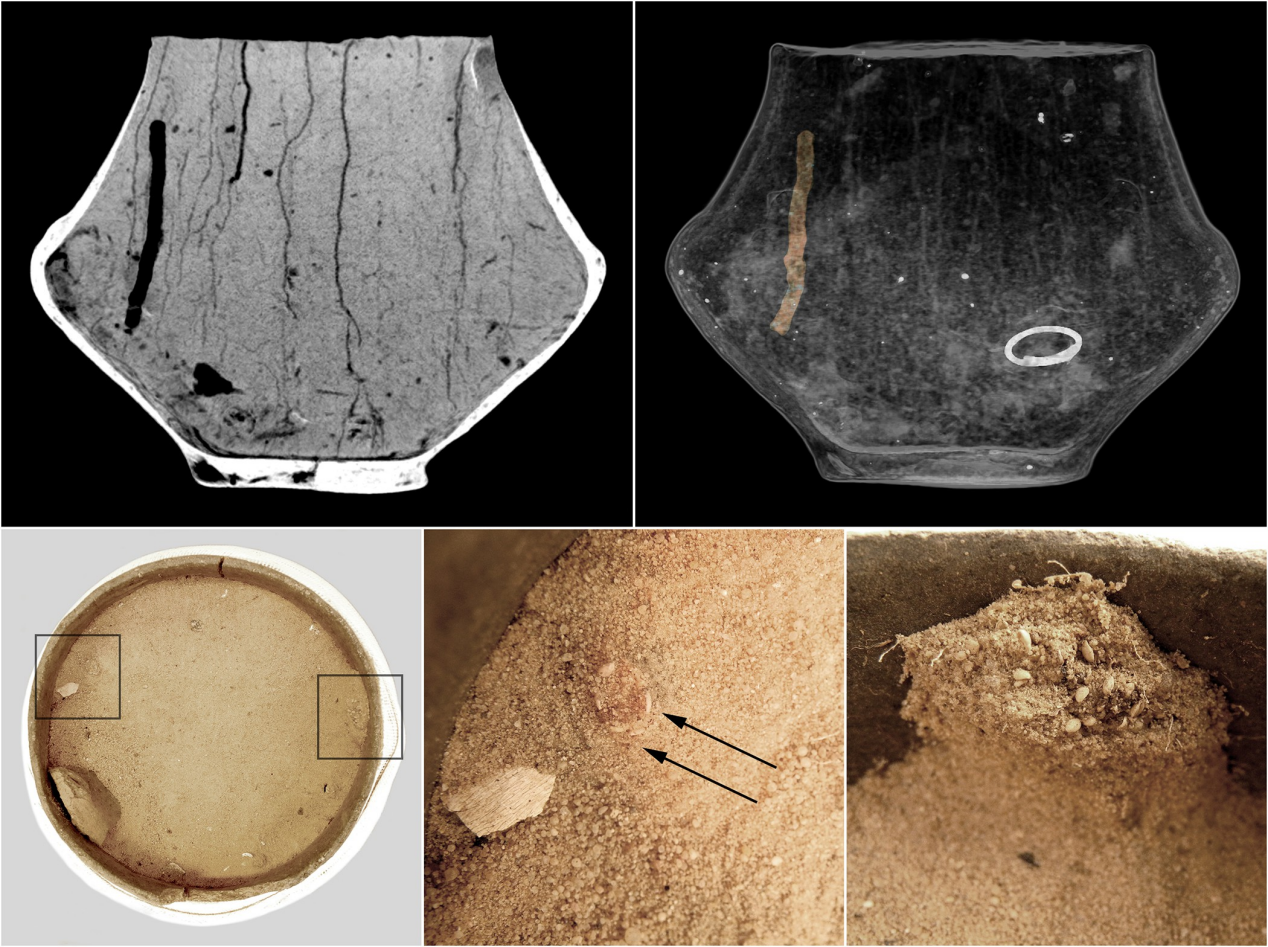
The CT imaging of the fill of the urn from grave 599. At the top, the MinIP and MIP reconstructions of the urn with a visible corridor of an insect of the genus *Harpalus* sp. At the bottom, the location of the corridor filled with *Setaria sp*. caryopses and the caryopses deposit identified during the spit exploration of the urn.
